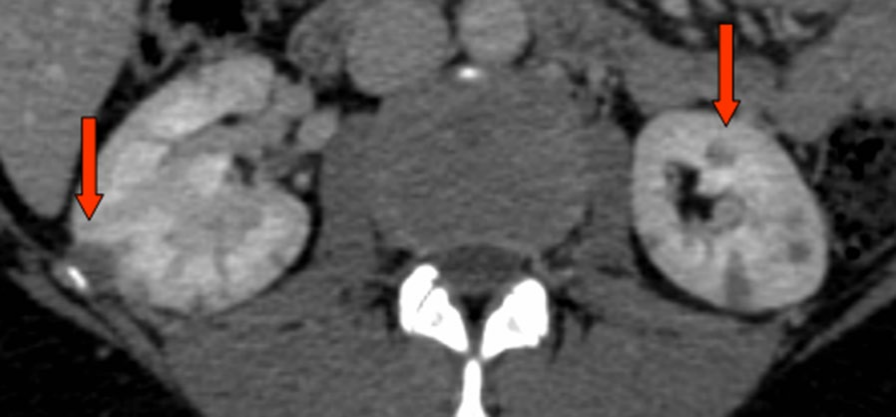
Contrast-enhanced CT-scan with detection of multiple, hypodense and bilateral kidney lesions
